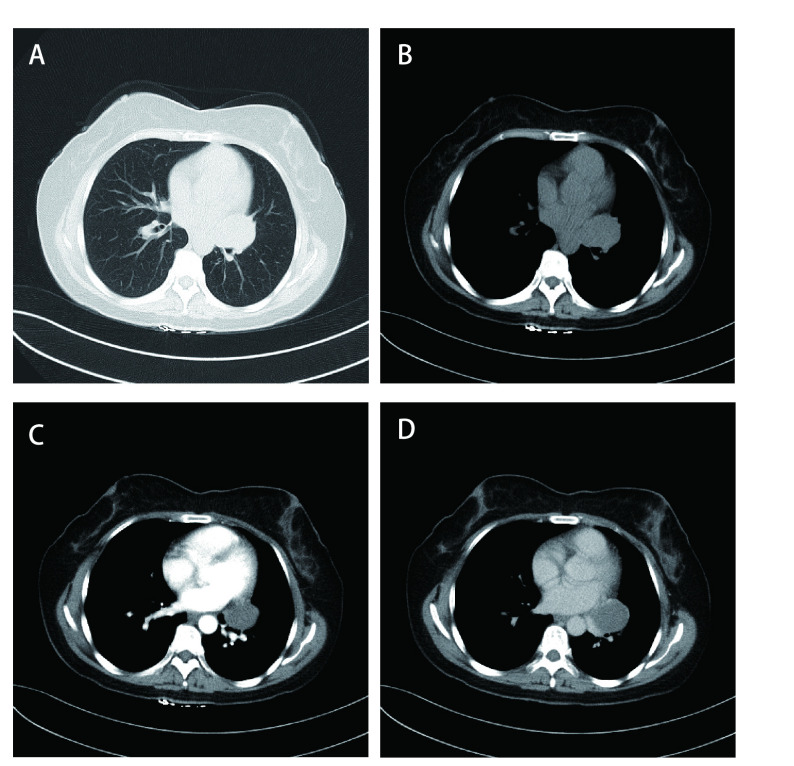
胸部CT：病变在肺门。A、B、C、D为同一患者：左肺门区见一密度增高团块影，边界较清。 Chest CT: lesions in the lung hilum. A, B, C and D are the same patient: A high density mass shadow was seen in the left hilar area, and the boundary was clear.
